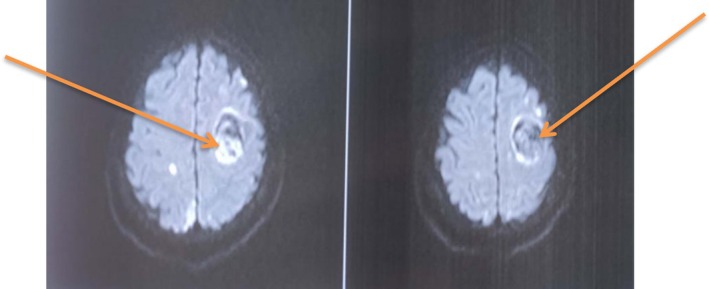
MRI brain DWI sequence shows left‐sided intracranial hemorrhage (orange arrows).
